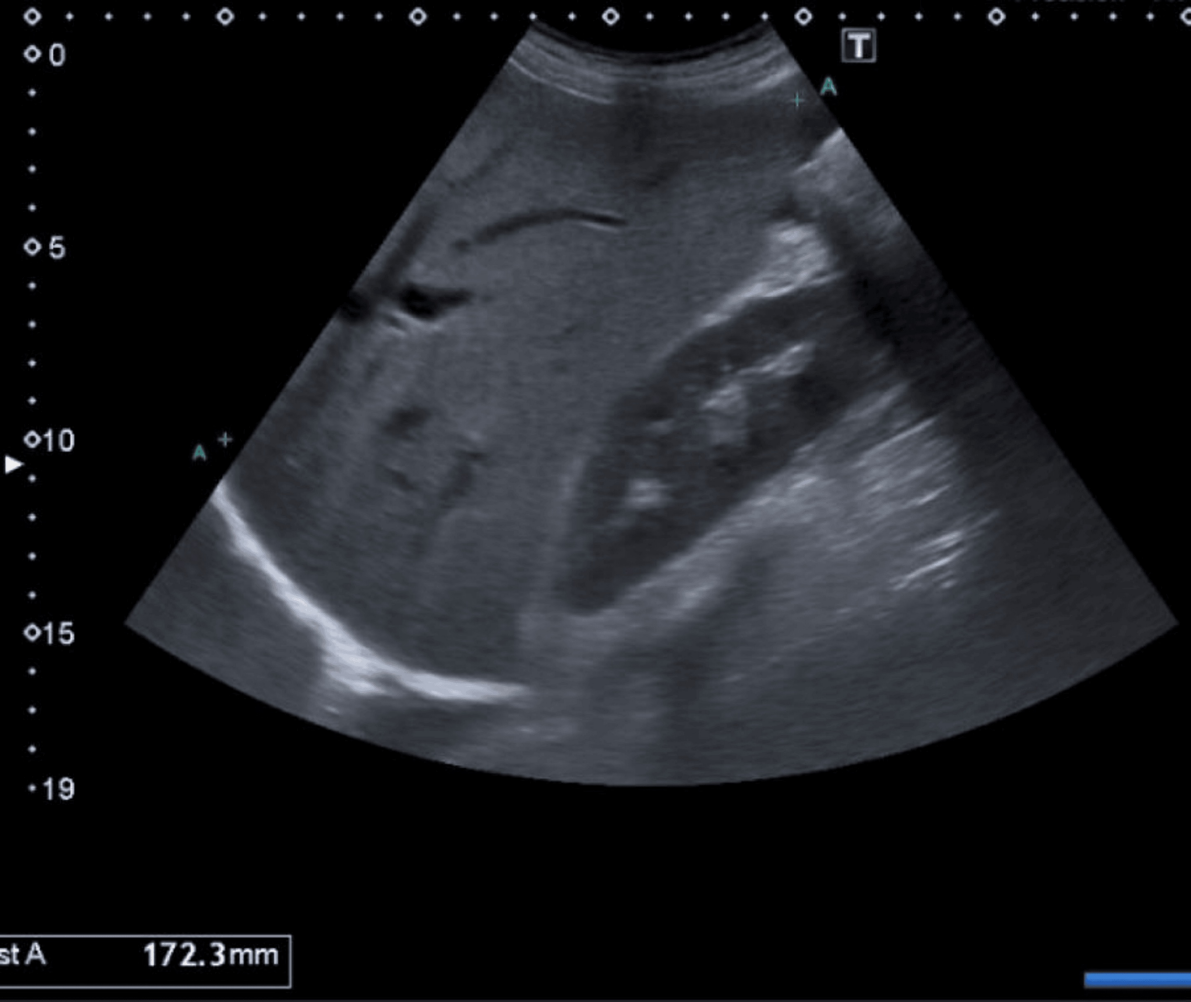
Hepatomegaly detected on abdominal ultrasound
